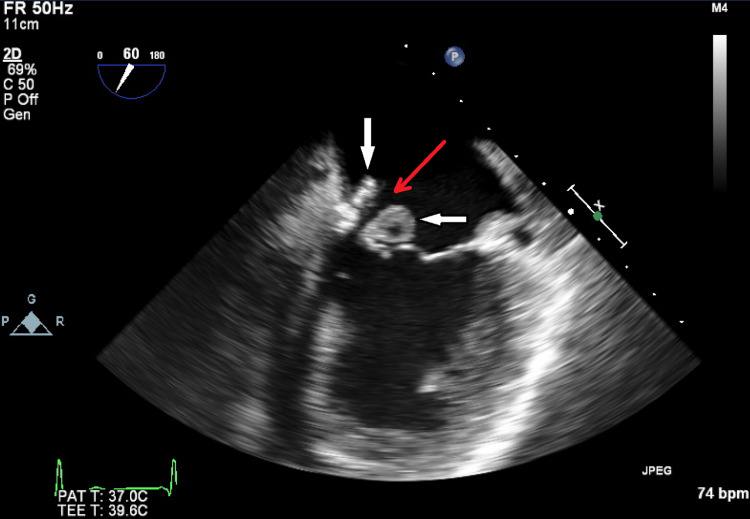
Transesophageal echocardiogram with a mid-position view displaying a vegetation (white arrows) on the mitral annulus with extension to the anterior and posterior mitral leaflets and perforation of the anterior mitral leaflet (red arrow)
